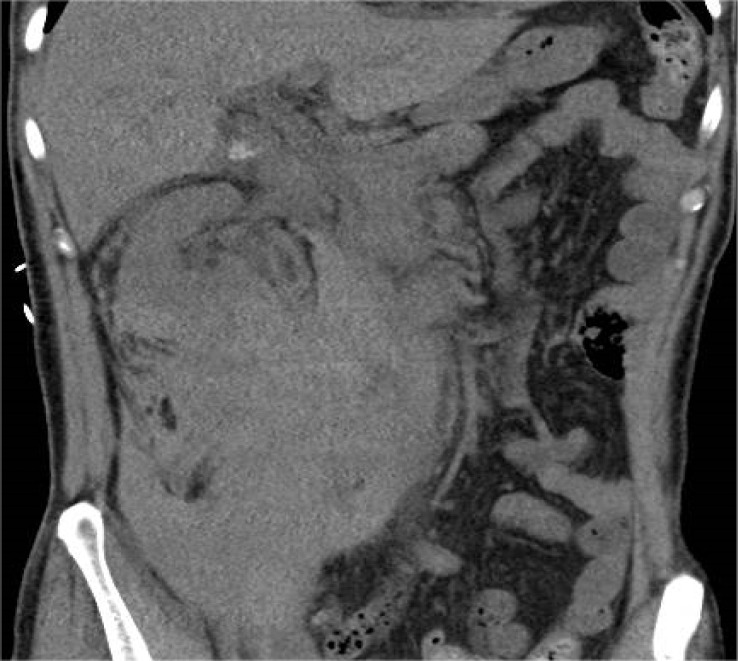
CT coronal section revealing a perirenal hematoma on the right kidney.
